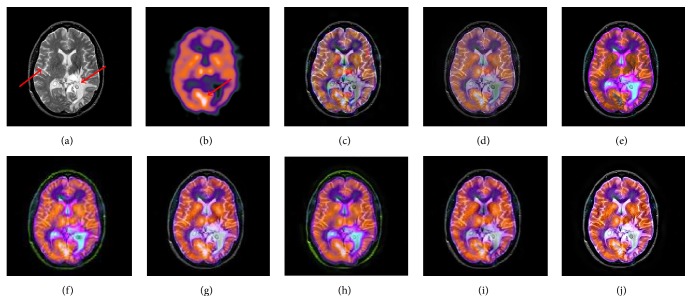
The fusion results of different fusion methods for the first set of MRI and SPECT images: (a), (b) Source images; fused images by (c) DWT, (d) GP, (e) IHS, (f) NSST-2, (g) NSST-3, (h) NSCT-3, (i) NSCT-4, and (j) the proposed method.
